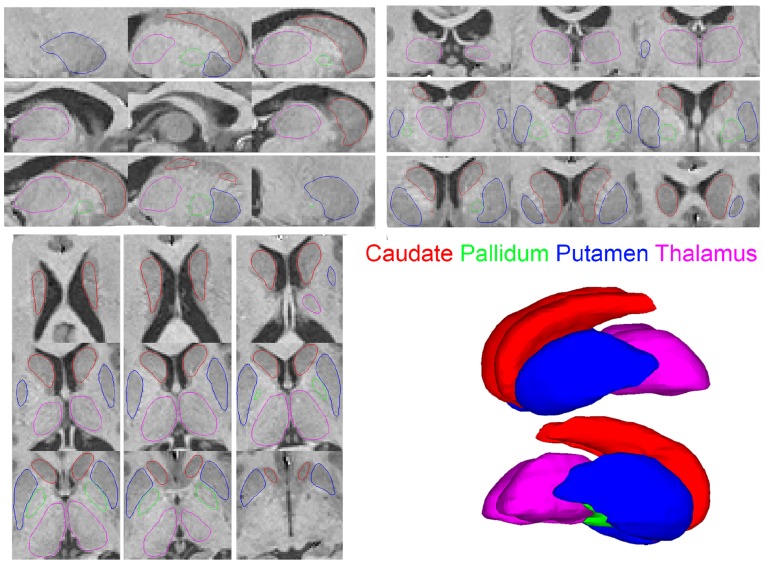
Saggital *upper left* Coronal *upper right* Axial *lower left* views of the segmentation outlines overlayed on the corresponding MRI slices. The 3D surface renderings show the smooth surfaces *lower right*.
